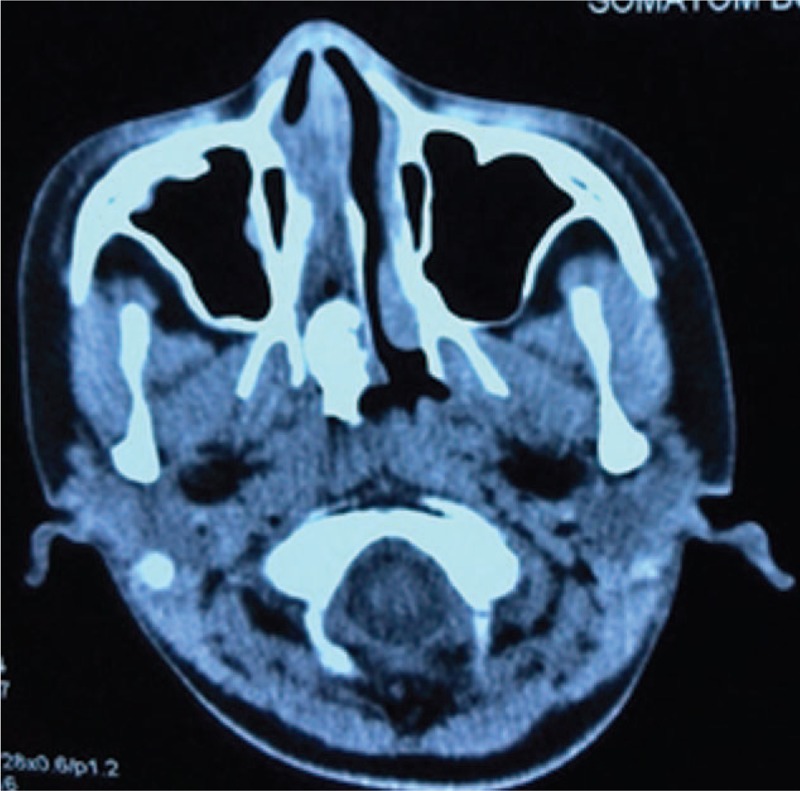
Axial CT of the paranasal sinuses showing the tumor mass affecting the right-sided nasal cavity and nasopharynx. A new bone-like material can be seen in the nasopharynx, without bony erosion involvement of the nasal septum, orbital, and skull base. CT = computed tomography.
